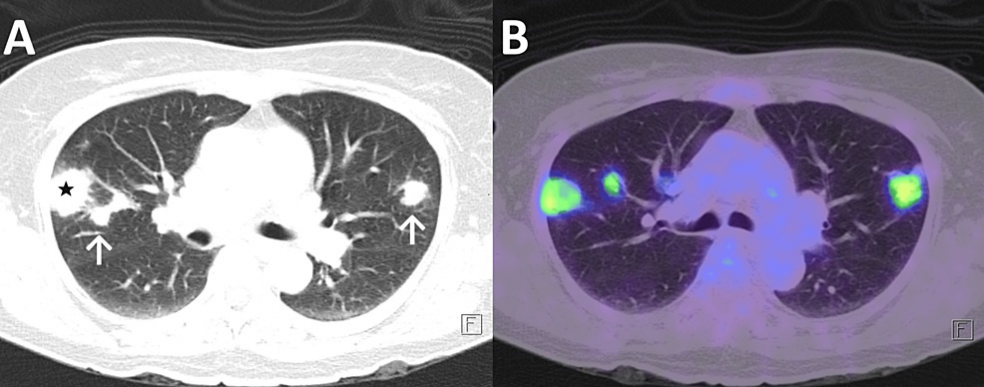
PET-CT. Pane A: Lung window showing right lung consolidating lesion (black star) with nodules in both lungs (white arrows). Pane B: PET window showing 18-fluorodeoxyglucose avidity in the lesion and nodules.
